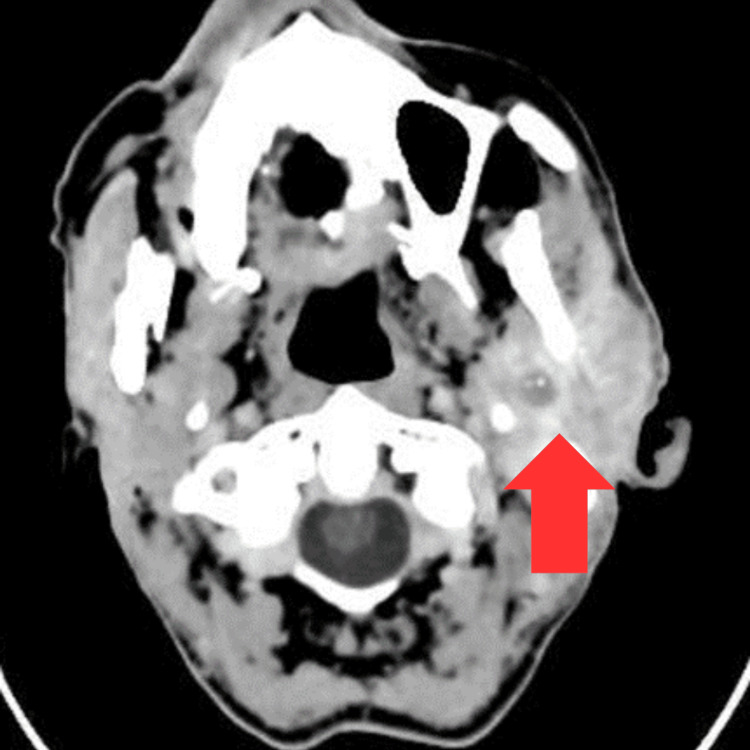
Brain CT post-contrast in mediastinum window showing a voluminous, ill-defined, heterogeneous soft-tissue mass of the left parotid gland, involving both the superficial and deep lobes and resulting in glandular enlargement CT: computed tomography
